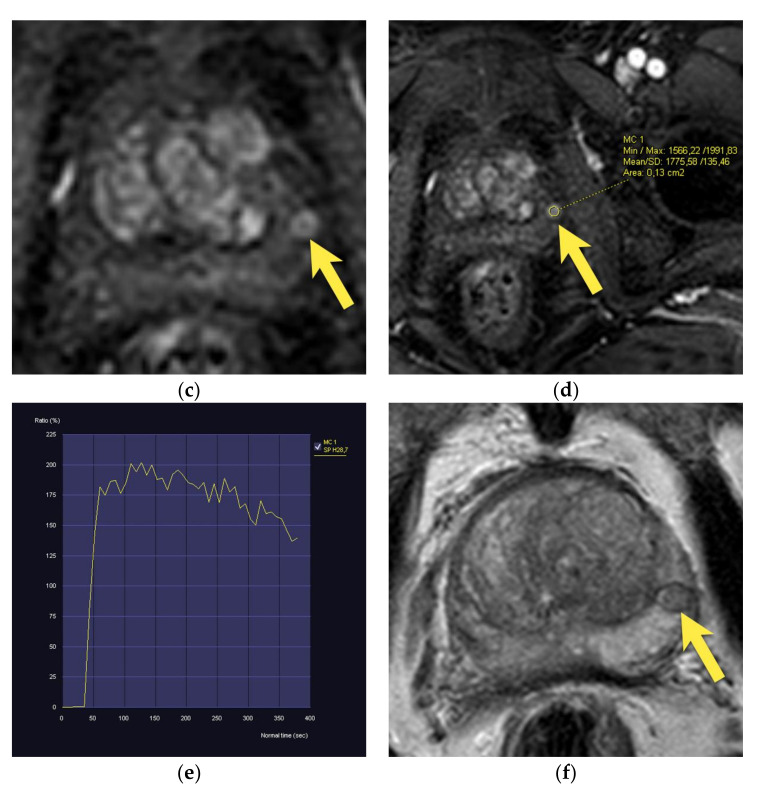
Ectopic BPH nodule in the left peripheral zone: focal lesion with marked diffusion restriction with (a) high signal on DWI b 2000 image (arrow); (b) low signal on ADC map (arrow); (c) early and strong enhancement on DCE; (d) ROI for calculation of enhancement curve placed in the lesion on DCE; (e) enhancement curve in the lesion shows strong, early enhancement with washout—only in these sequences the lesion appear suspicious for malignancy. However, T2-weighted image (f) revealed completely encapsulated BPH nodule, which should be scored as benign—PI-RADS 1.
